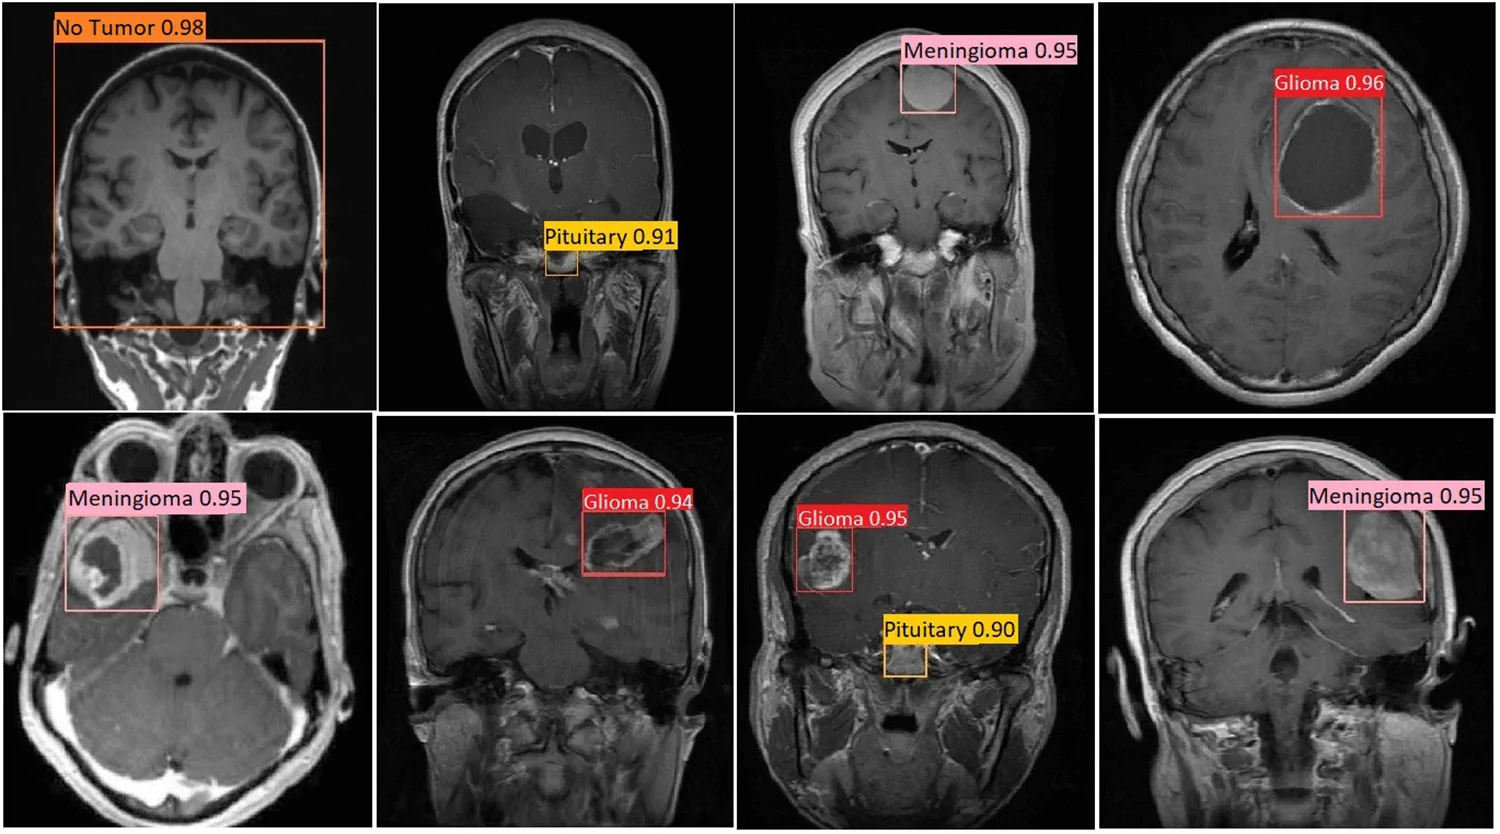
RF-DETR(small) prediction results. The underlying base MRI scans are obtained from the “Brain Tumor MRI” open-source dataset by workspace research-jz5sv [21] on Roboflow Universe, used under the CC BY 4.0 license.
